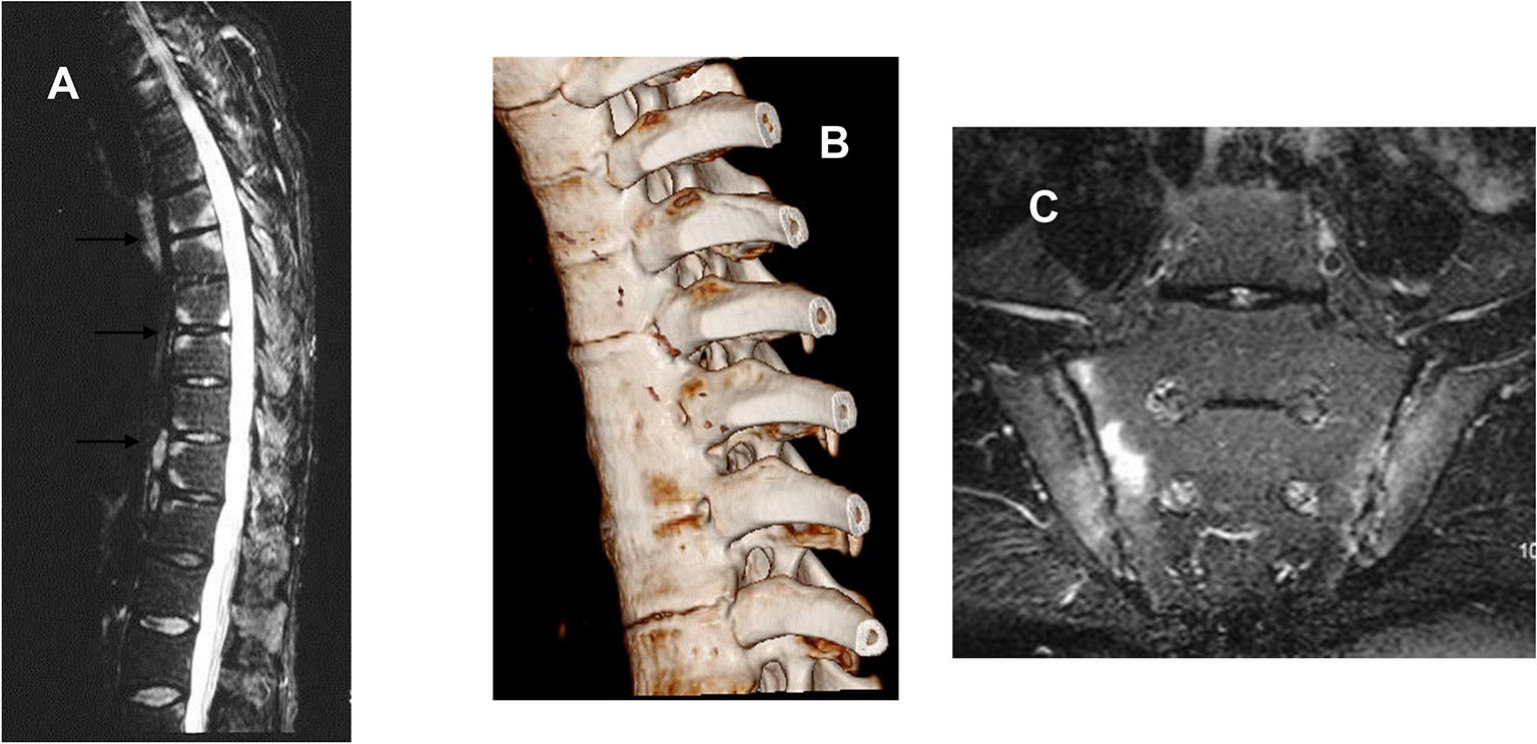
(A) Sagittal magnetic resonance image of the thoracic spine of a 44-year-old man with active ankylosing spondylitis, showing high signal on these T2-weighted images consistent with inflammation at the vertebral corners consistent with the attachment of vertebral ligaments and discs. (B) Computed tomographic reconstruction of the thoracic spine of a 25-year-old man with AS since the age of 12. There is clear bony fusion between the adjacent vertebrae and also at the costovertebral joints. (C) Bilateral sacroiliitis shown by MRI (STIR sequence) worse on the sacral side of the right SI joint.
